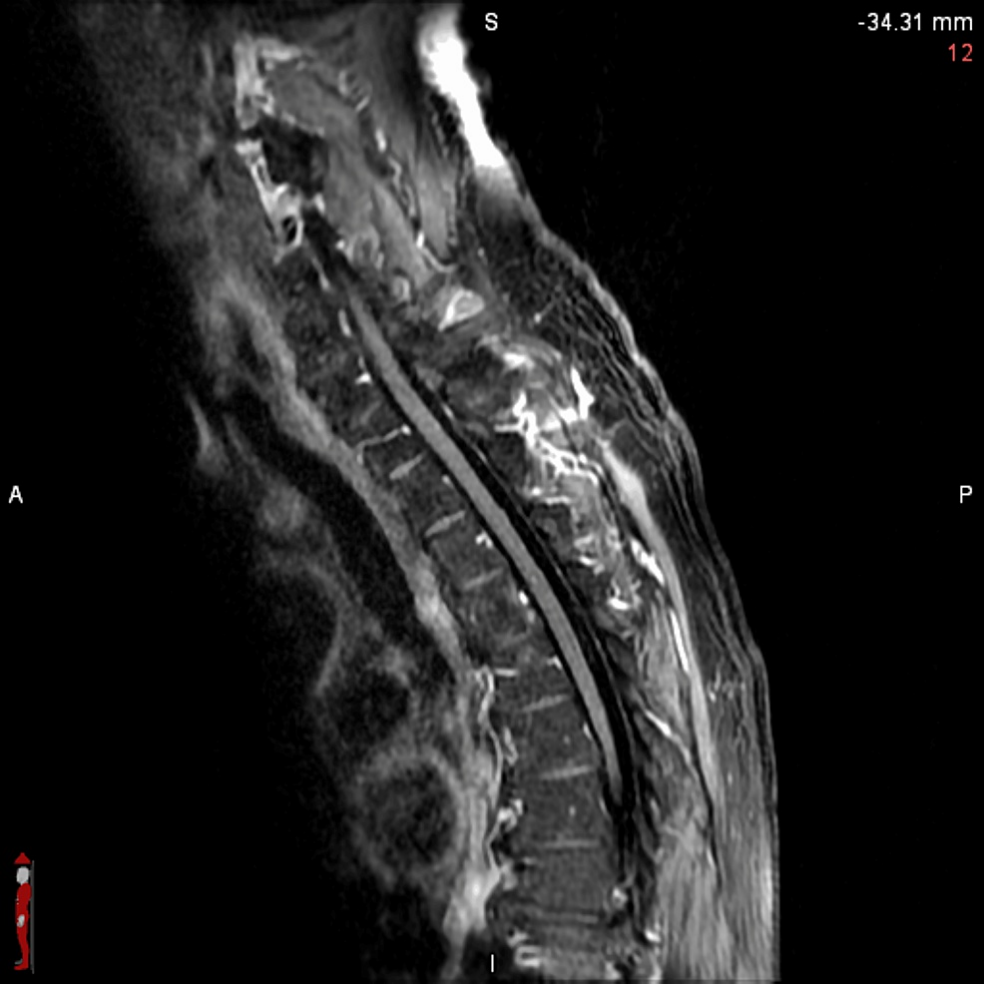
Sagittal spine MRI T1 FLAIR-FS from C2 to T9 showing no abnormal enhancement or signs of leptomeningeal disease FLAIR-FS: fluid-attenuated inversion recovery with fat suppression
